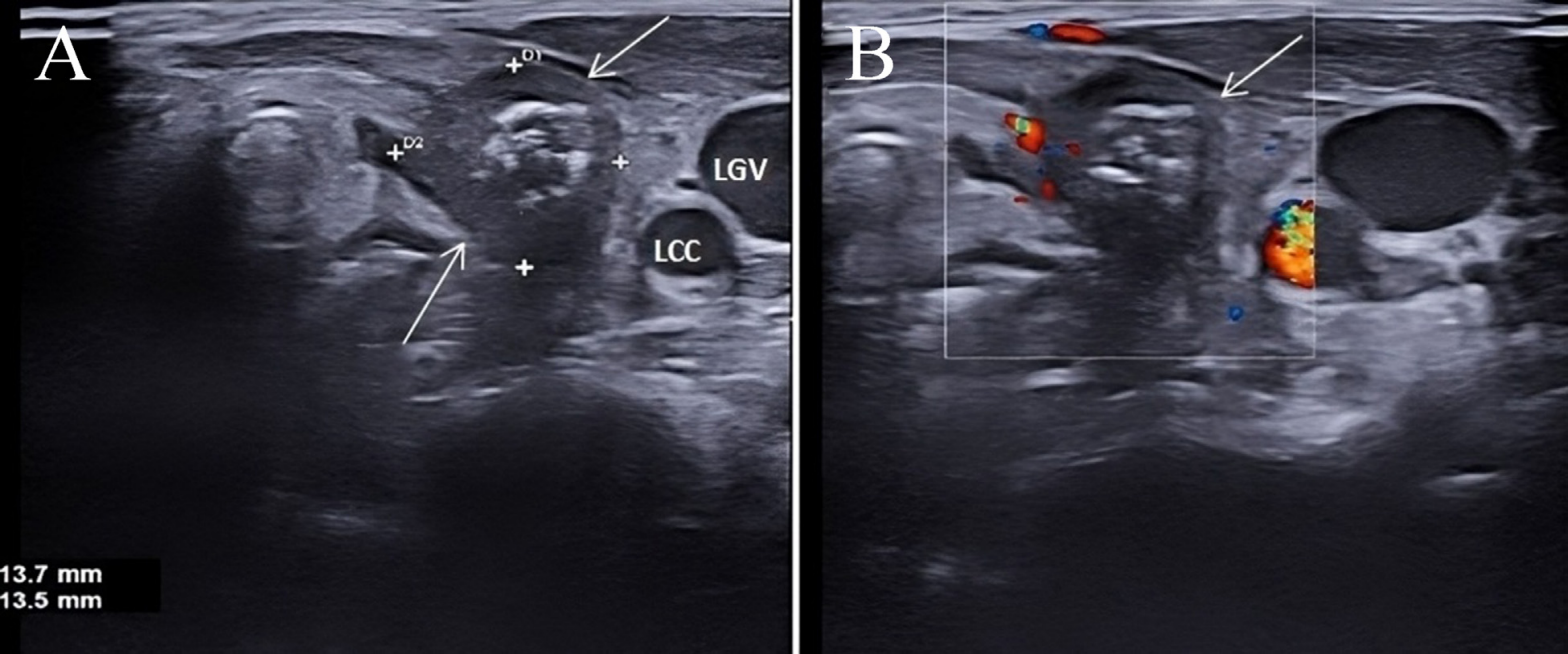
Us of the neck. Adjacent to the left thyroid lobe, gray-scale scans show (A) a rounded fluid mass with aerial content (arrows) that with (B) the color Doppler US does not shows intra -lesional vascular signals but only peripheral vascular signals that make suspects the ascessual nature of the lesion.
